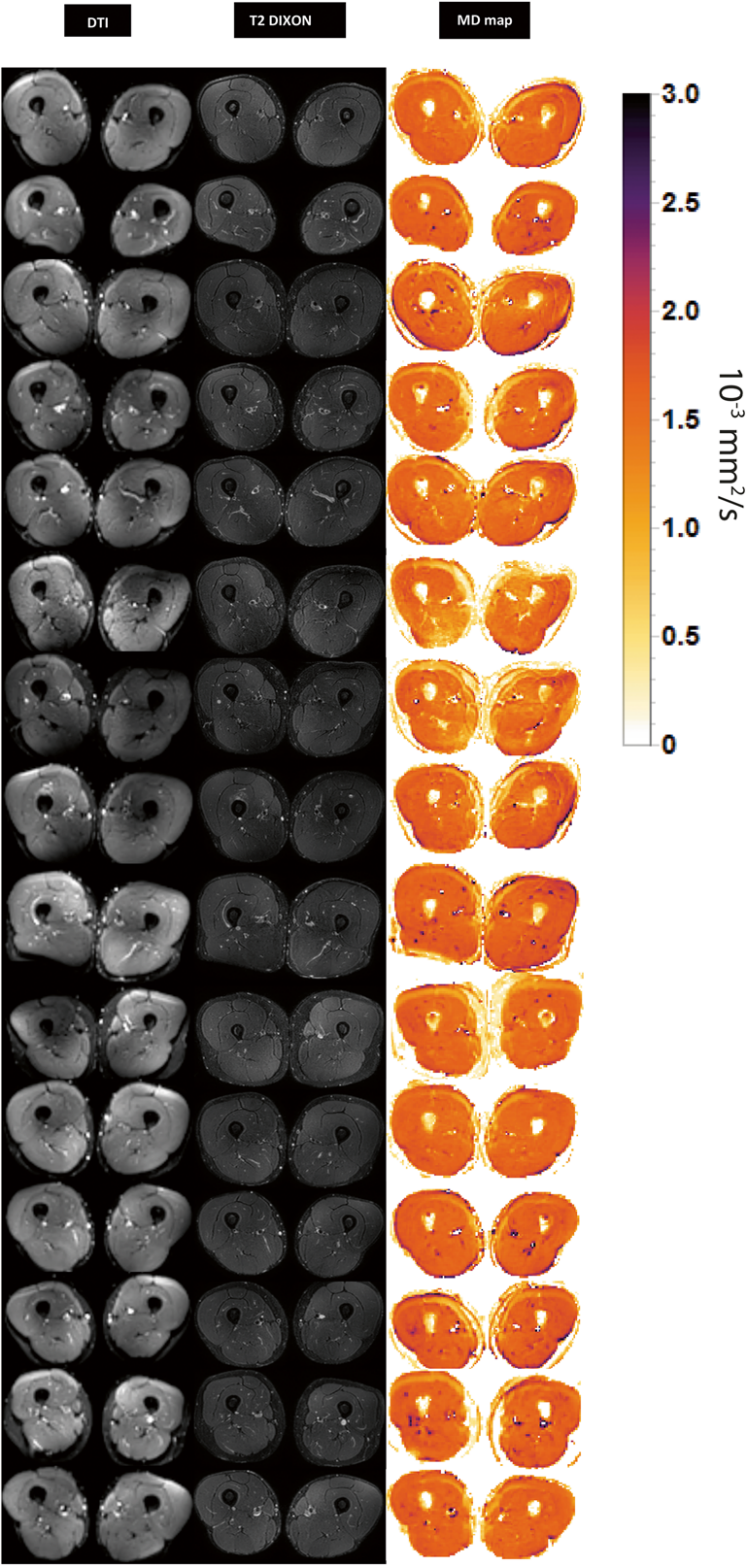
Representative axial slice of the DTI (*b* value 0 s/mm^2^) images, T2-weighted images, and MD maps for all 15 subjects at the first time point
